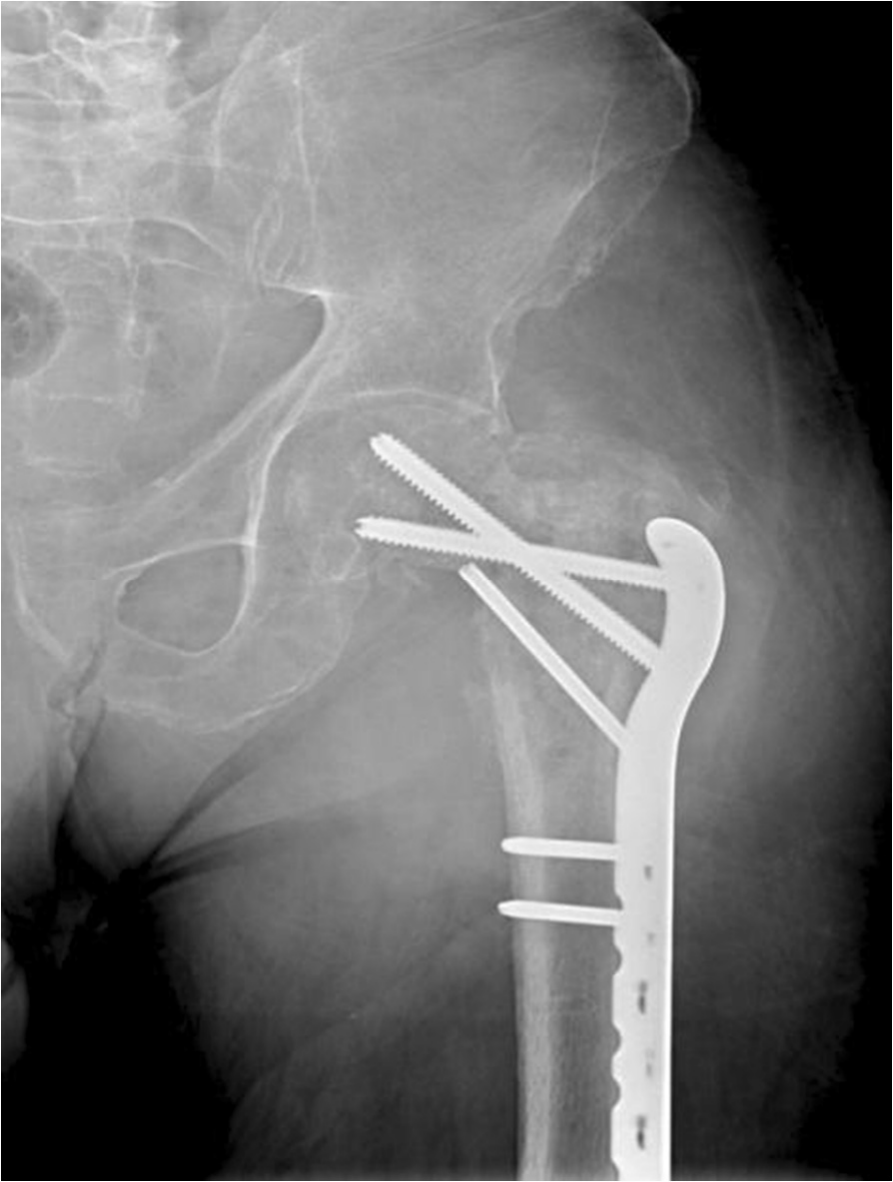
Anterior-posterior radiograph of the left hip. This shows a proximal femoral locking plate in good position with moth-eaten appearance of proximal femur and surrounding lysis that could be suggestive of infection or malignancy.
